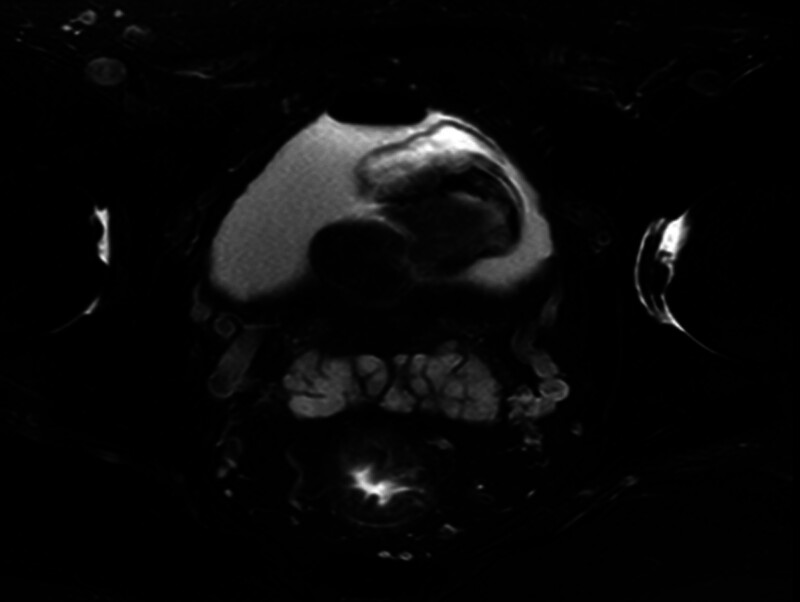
Pelvic MRI: an irregular, mass-like abnormal signal is seen within the bladder cavity.
